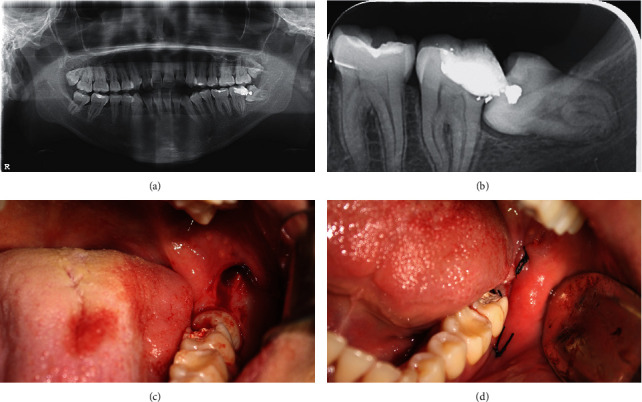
Surgical extraction of mandibular left 3^rd^ molar for Case 1. Presurgical evaluation included panoramic (a) and periapical (b) radiographic assessment in addition to clinical examination. The procedure was started by a pyramidal flap incision followed by mucoperiosteal flab elevation (c). Next, bone removal to expose the impacted molar was completed as needed followed by guttering buccally and distally. The site was sutured with simple interrupted sutures using 3-0 silk and hemostasis achieved (d).
